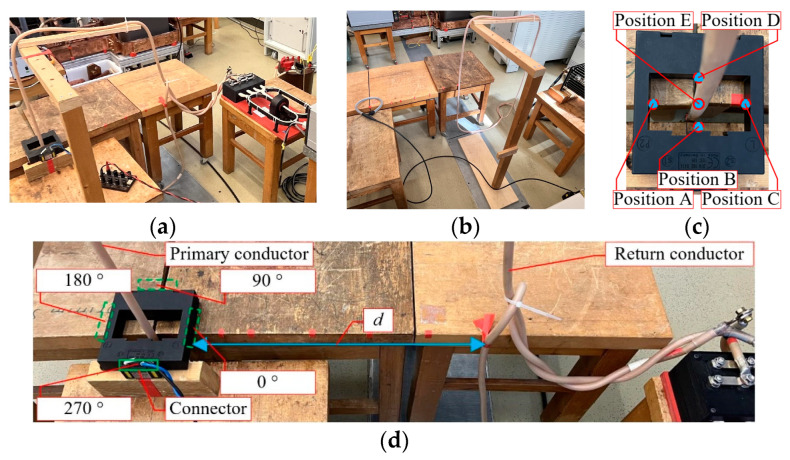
Photos of laboratory test setups for the considered influence quantities: (a) measurement setup for position effect, (b) measurement setup for adjacent effect, (c) position of centering, (d) return conductor effect as a function of the CT connector position.
